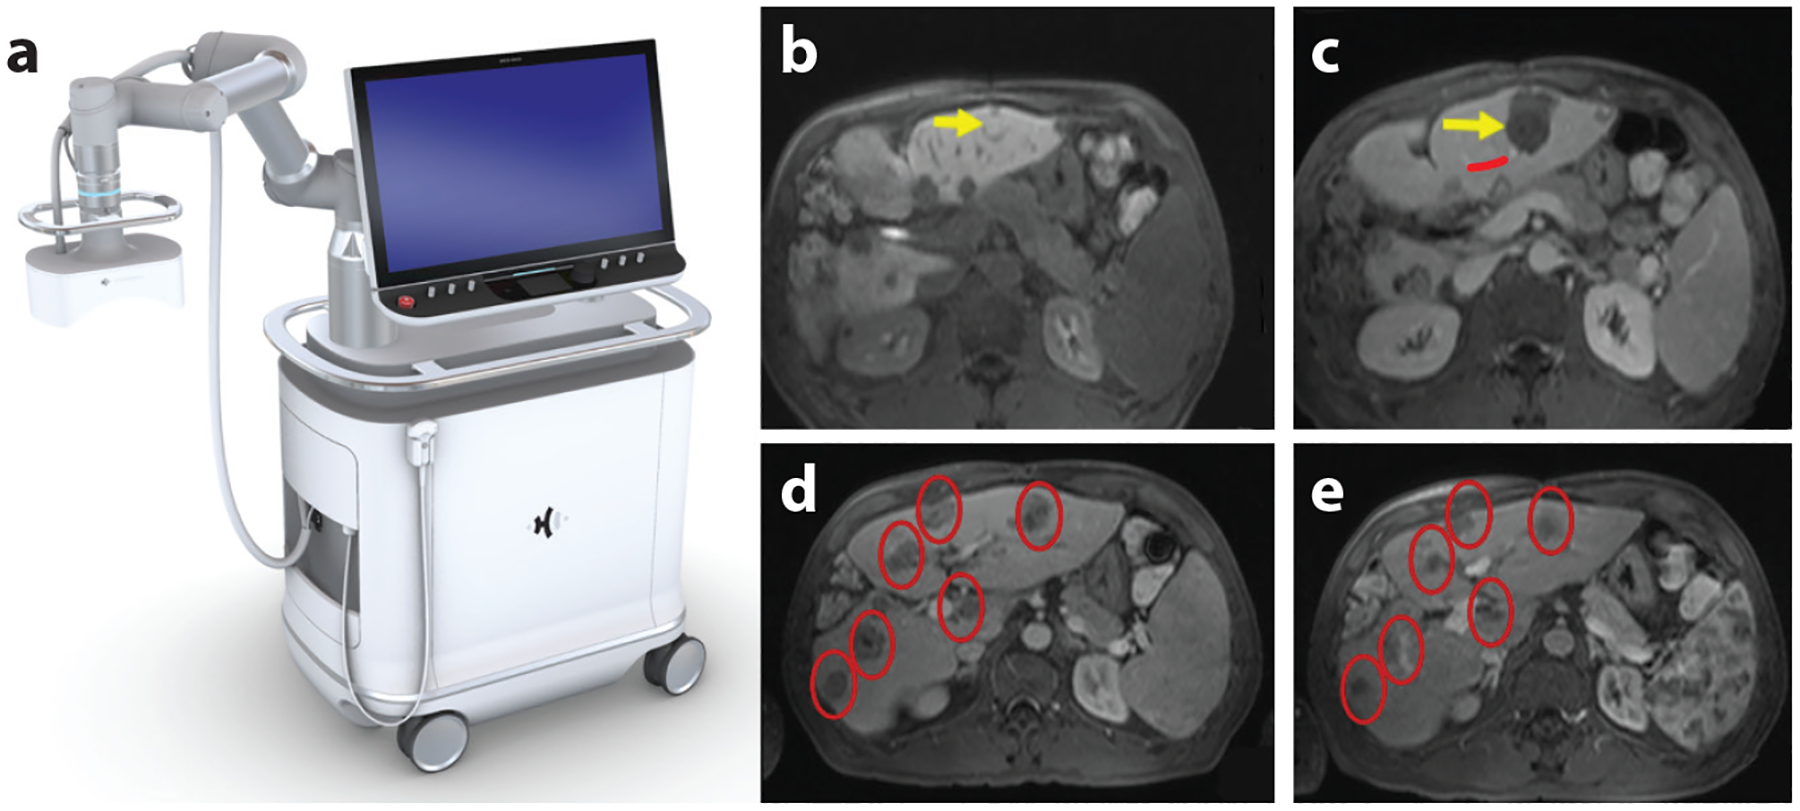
Results from clinical trials. (*a*) An image of the ultrasound image–guided clinical prototype histotripsy system (Edison^™^ platform; HistoSonics, Inc.). (*b*–*e*) Magnetic resonance (MR) images from the THERESA histotripsy liver tumor clinical trial (51). (*b*,*c*) MR images of the targeted tumor 5 mm adjacent to the hepatic vessel branch (*b*) before and (*c*) after shock scattering cavitational histotripsy, showing effective ablation of the liver tumor (tumor indicated by the *yellow arrow* in panel *b*) with a margin (ablation indicated by the *yellow arrow* in panel *c*) and intact hepatic vessel (indicated by the *red line* in panel *c*). (*d*,*e*) MR images of nontargeted tumors (*red ovals*) (*d*) before and (*e*) 8 weeks after histotripsy treatment, showing the abscopal response as a size reduction.
